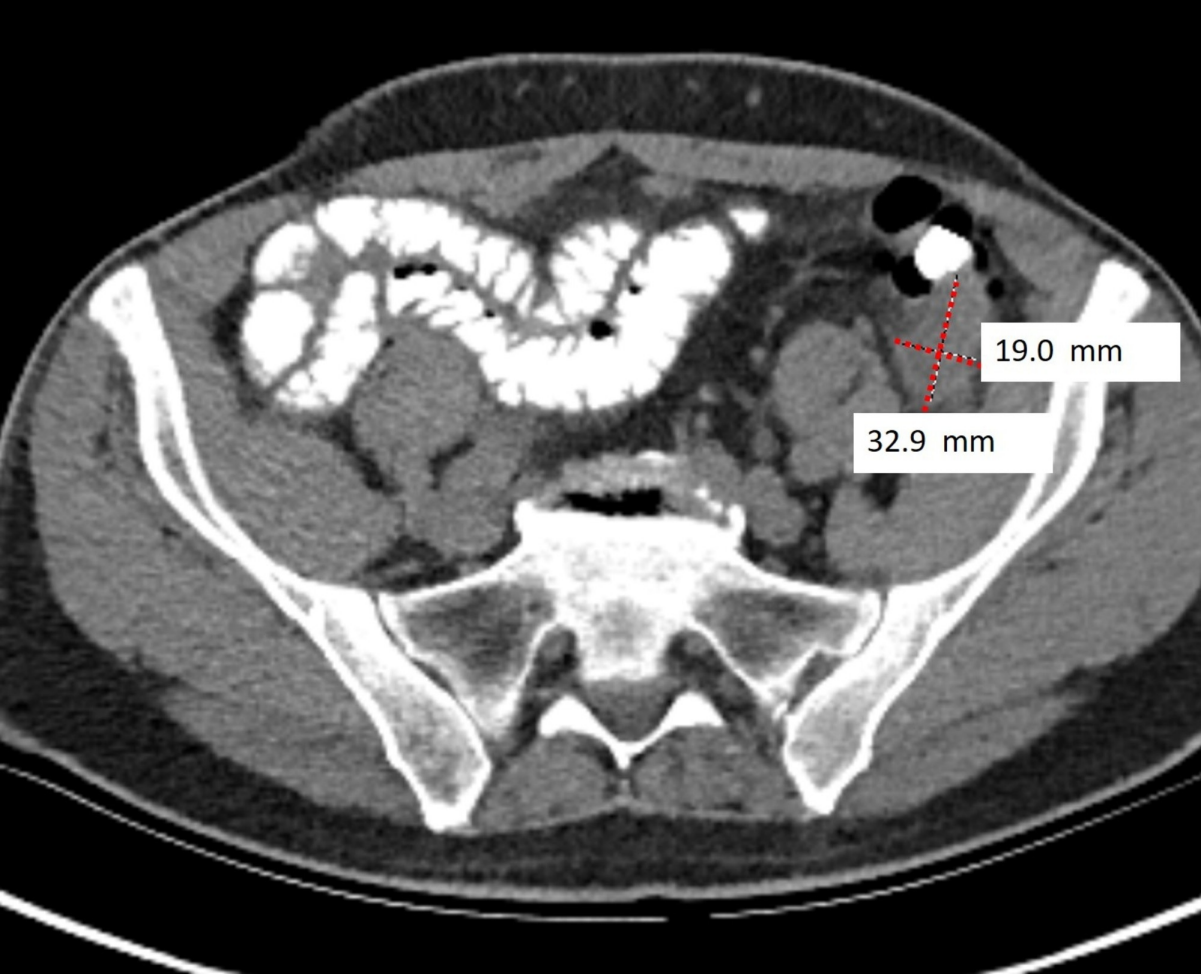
Repeat CT scan showing a peritoneal mass, thought to be postinflammatory process (diverticulitis).
